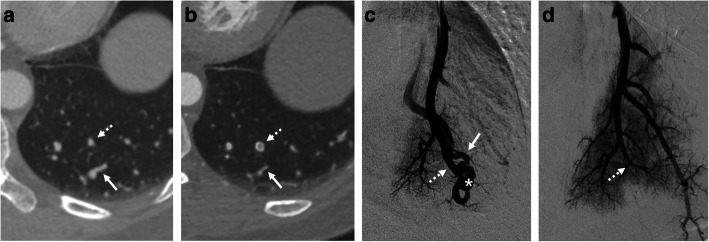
A 40-year-old female who underwent embolization 41 months earlier with a simple PAVM of the left lower lobe. Arrows, dashed arrows, and asterisks indicate draining vein, feeding artery, and venous sac, respectively. CT images obtained before (a) and after (b) embolization. The draining vein decreased from 2.4 mm to 1.1 mm after embolization (54.2 % reduction), and a vascular plug was deployed in the feeding artery. Occlusion was confirmed by pulmonary angiography obtained before (c) and after (d) embolization
